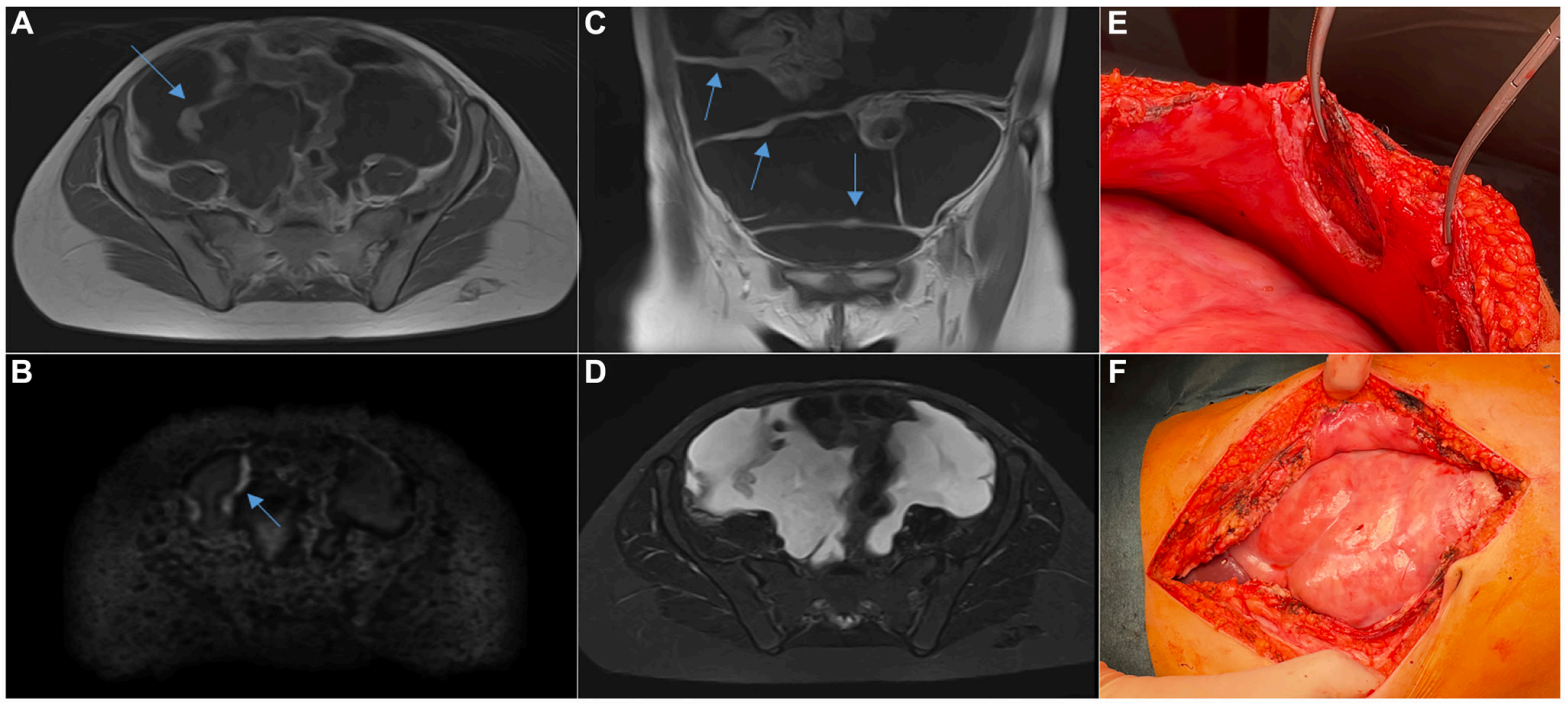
Peritoneal carcinomatosis during disease progression on immunotherapy. (A) T1 weighted image (WI), contrast-enhanced, axial plane; (B) DWI (b = 1000 c/mm^2^), axial plane; (C) T1WI contrast-enhanced, coronal plane; (D) T2WI STIR, axial plane; uneven thickening of the peritoneum against the background of ascites, visualized by contrasting, restricted diffusion; (E) intraoperative biopsy of the thickened peritoneum; (F) intraoperative view of the abdominal cocoon-encapsulated colon.
